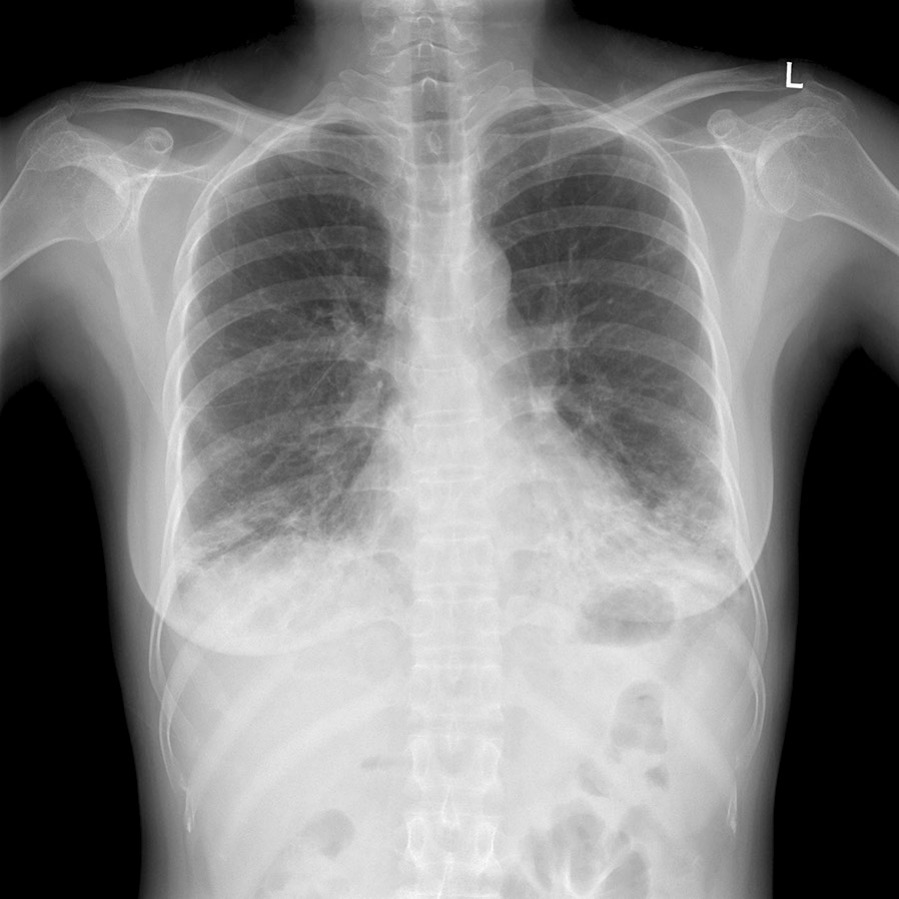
Preoperative chest radiograph. A chest radiograph showed an increased reticular opacity at both lower lung zone in preoperative evaluation
